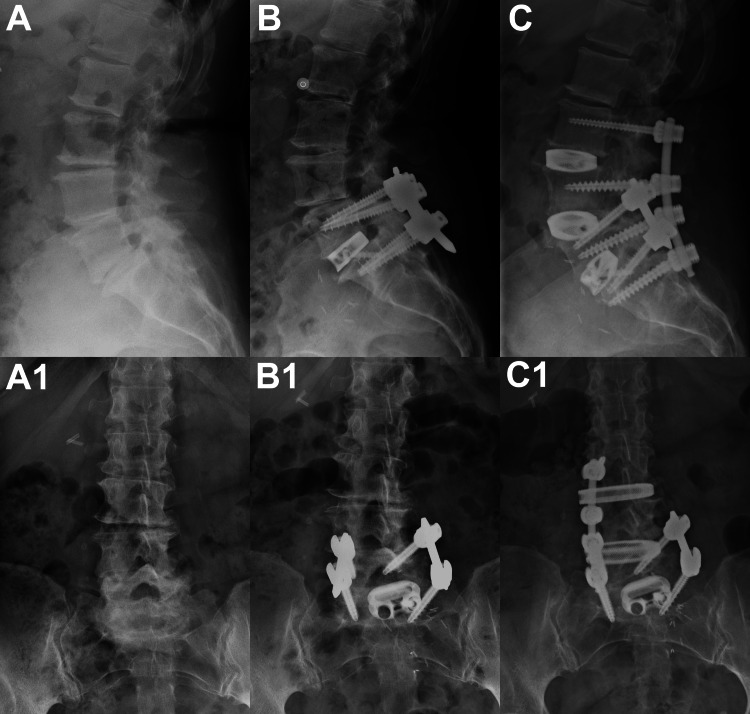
Radiographs of our patient's lumbar spine surgeries. Lateral (A) and anterior-posterior (A1) radiographs taken a few weeks before our patient’s first lumbar spine surgery. Lateral (B) and anterior-posterior (B1) radiographs after the second lumbar spine surgery, which included L5-S1 interbody fusion with posterior instrumentation using pedicle screws and left and right rods. Lateral (C) and anterior-posterior (C1) radiographs of the third (and final) lumbar spine surgery (November 2022), which included L3-L4 and L4-L5 interbody fusions with posterior instrumentation using pedicle screws and rods (long on the left and short on the right).
